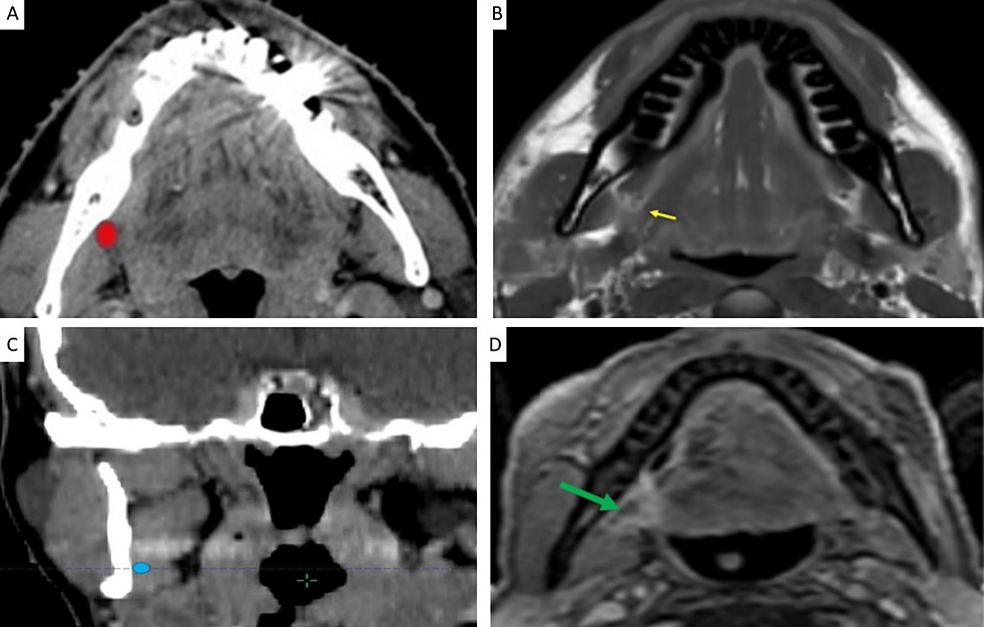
Anchor point at the level of oral tongue A: Location of the lingual nerve (red) located at the inferior pterygomandibular space is located between the anterior margin of the medial pterygoid muscles and the posterior free margin of the mylohyoid muscle B: Location of the lingual nerve (yellow arrow). Axial non-contrast T1W MR shows the lingual nerve located between the anterior margin of the medial pterygoid muscle and the posterior margin of the mylohyoid muscle C: Location of the lingual nerve (blue) on corresponding coronal CT slice D: Local failure in the lingual nerve pathway (green arrow). Fat-suppressed contrast-enhanced T1W sequence shows abnormal enhancement and thickening of the lingual nerve at the expected location
